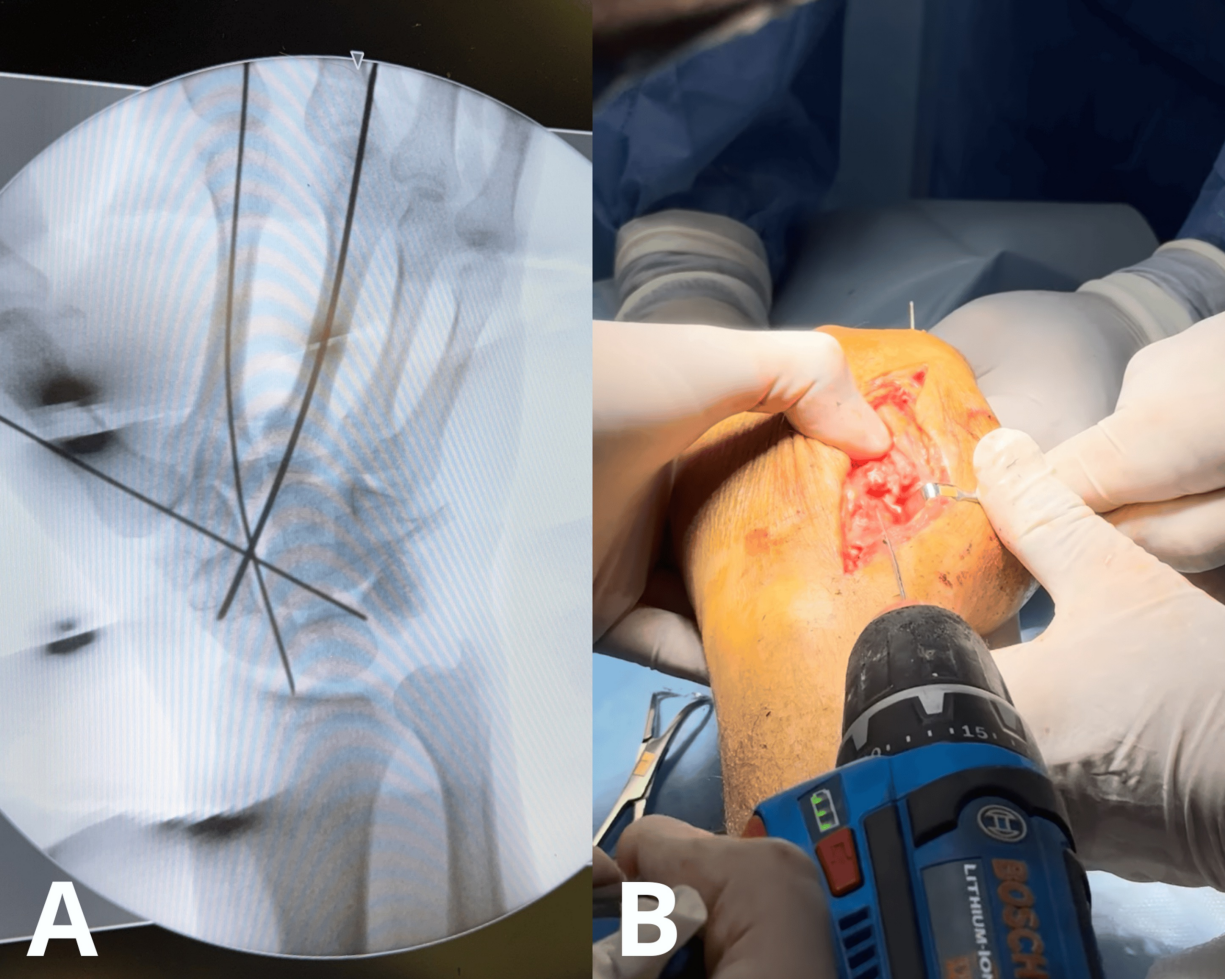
Intraoperative Kirschner wire fixation (A) Intraoperative radiograph after placement of Kirschner wires demonstrating stabilization of the trapeziometacarpal joint. (B) Placement of Kirschner wires to restore proper alignment of the carpometacarpal joint.
